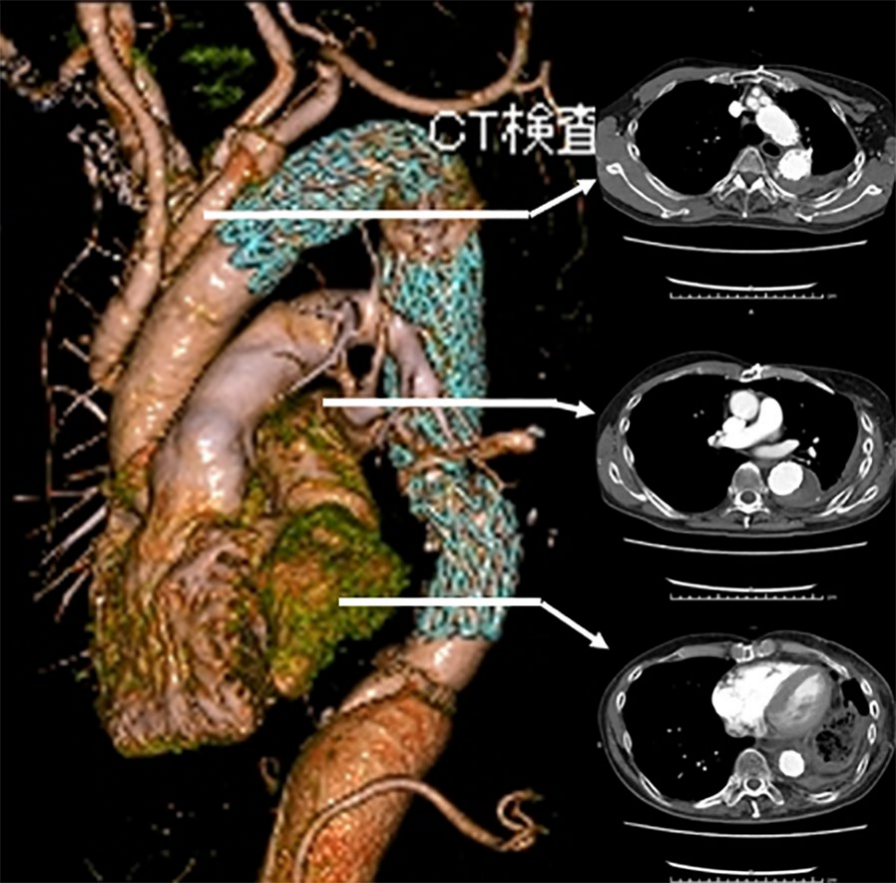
Enhanced computed tomography imaging. A postoperative CT scan did not show signs of further enlargement of the descending thoracic aorta with a completely thrombosed false lumen
